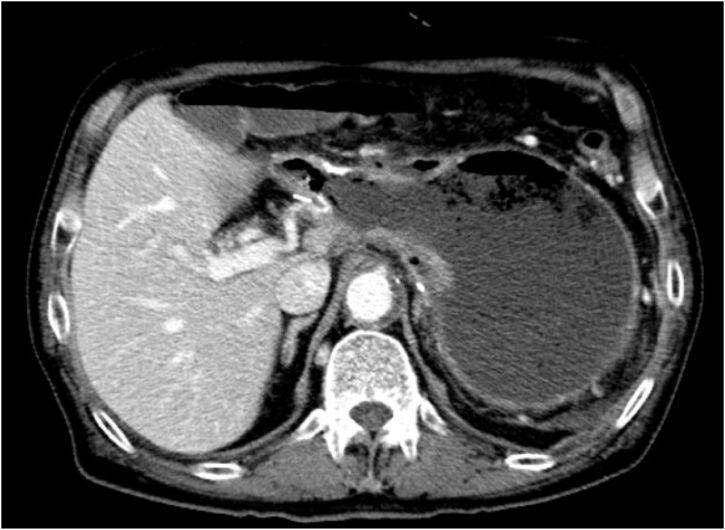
Axial computed tomography scan of the abdomen suggesting a high possibility of gastric ischemia and some fluid around the stomach.
